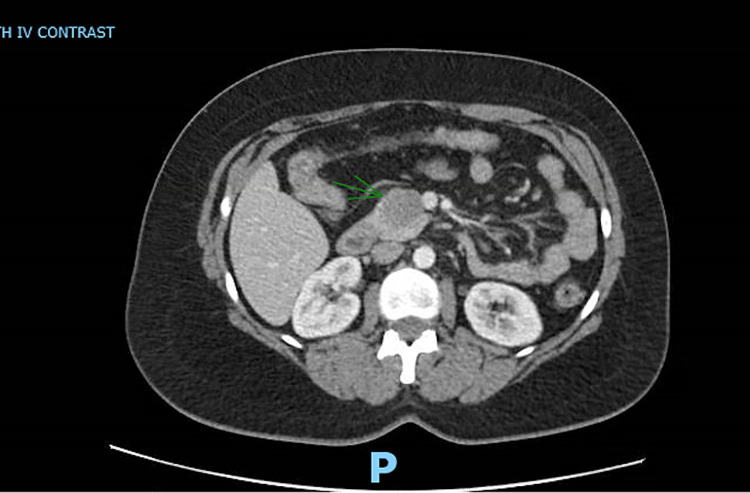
CT scan of the abdomen with intravenous contrast An axial section image of CT abdomen with intravenous contrast showing a 3.6-cm well-demarcated lobular hypoattenuating mass in the uncinate process of the pancreas (arrow) CT: computed tomography
